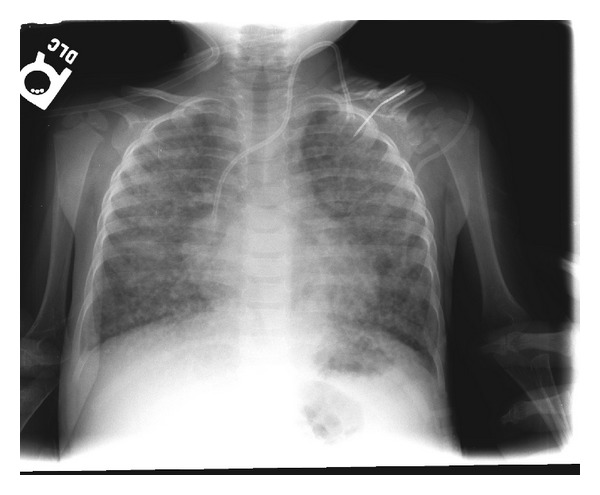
PA radiograph on admission: well-inflated lungs with diffuse bilateral coarse reticular opacities and left IJ port.
